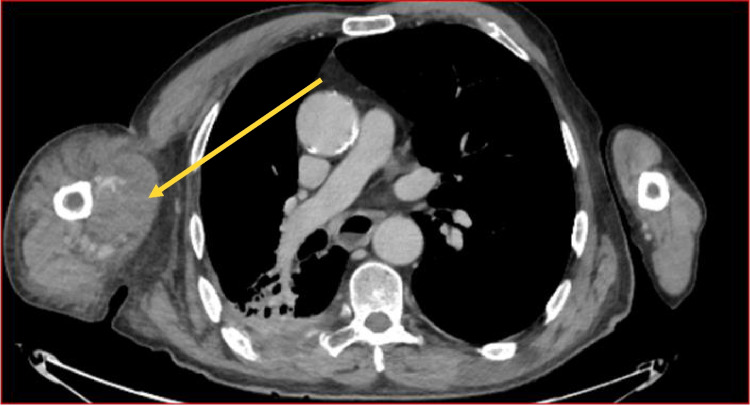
CT showing a hematoma of the right upper limb
